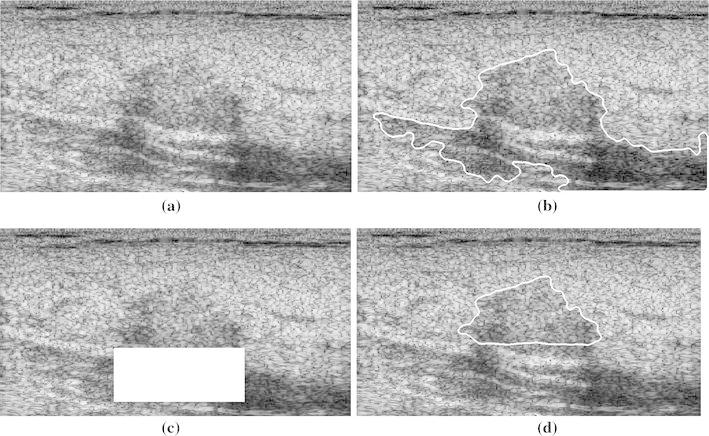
a Breast ultrasound image. b Tumor full contour extracted using adaptive thresholding and DE. c PAS part excluded to detect half contour. d Detected tumor half contour
